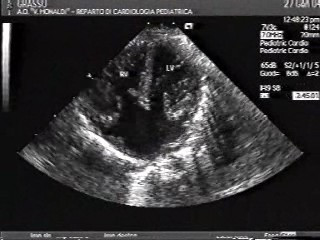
Echocardiography of Complete atrioventricular canal.
